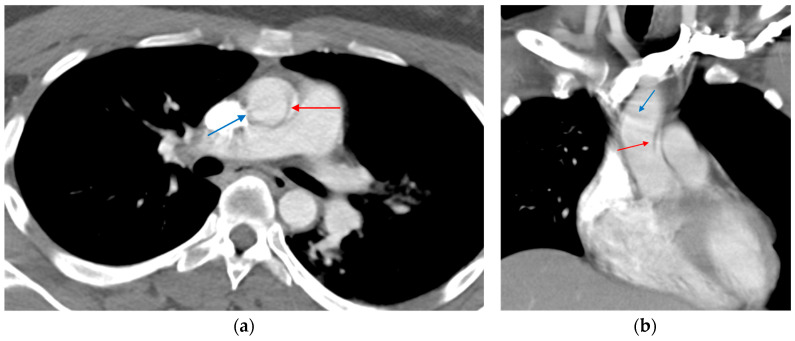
Pulsation artifact and streak artifact related to contrast beam hardening. (a) Axial and (b) coronal CTA images of the chest demonstrate a curvilinear intraluminal filling defect in the ascending aorta (red arrow), compatible with pulsation artifact and mimicking an intimal flap. Dense contrast within the superior vena cava produces an adjacent streak artifact (blue arrow) that further degrades the evaluation of the ascending aorta.
